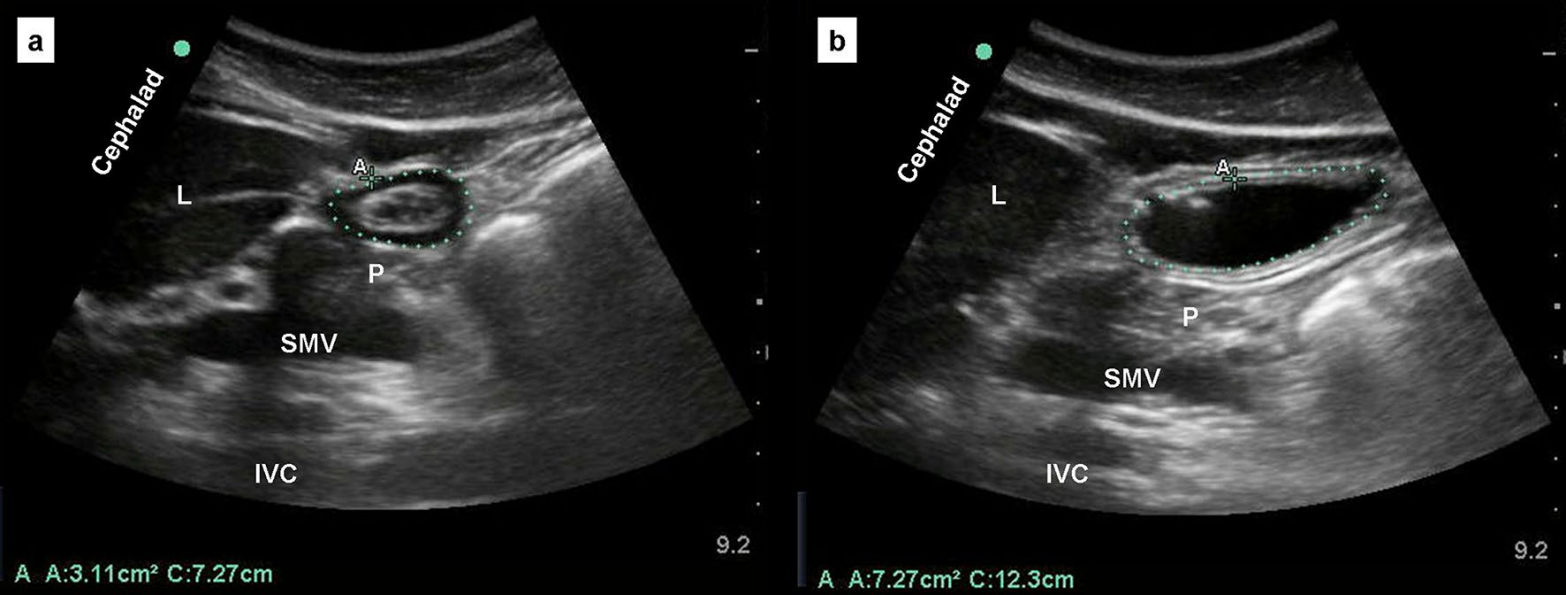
Antral ultrasound views from the same patient in the semirecumbent position (a) before and (b) after endoscopic endonasal transsphenoidal pituitary surgery. L: liver; P: pancreas; SMV: superior mesenteric vein; IVC: inferior vena cava. Dotted circle: gastric antrum.
